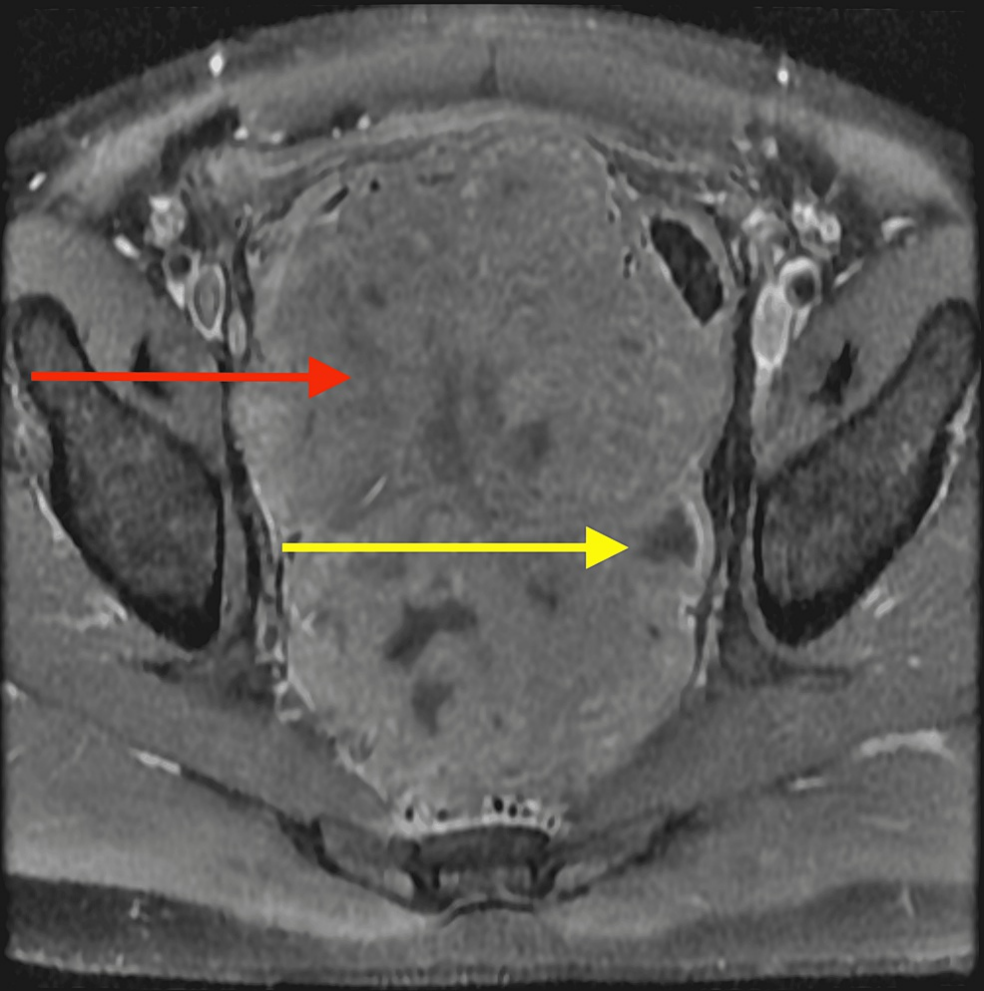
Axial MRI T1 post-contrast sequence shows an avidly enhancing heterogeneous mass (red arrow) with multiple areas of necrosis (yellow arrow)
